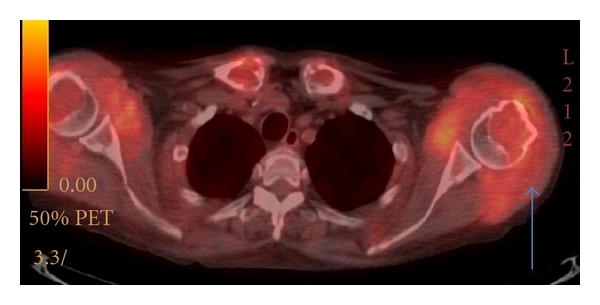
Axial fused PET/CT at the level of T2 vertebra: extensive FDG-avid biopsy-proven amyloid left deltoid muscle in a patient with multiple myeloma (arrow).
